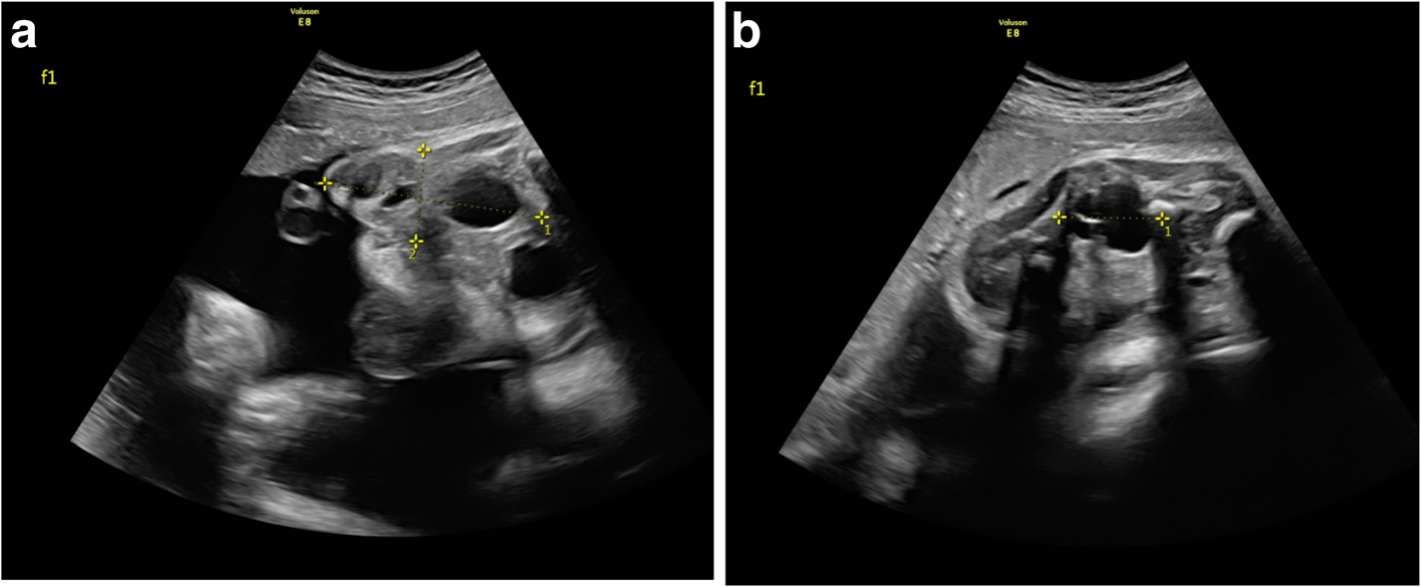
a, b Ultrasound view of the SCT in twin B
